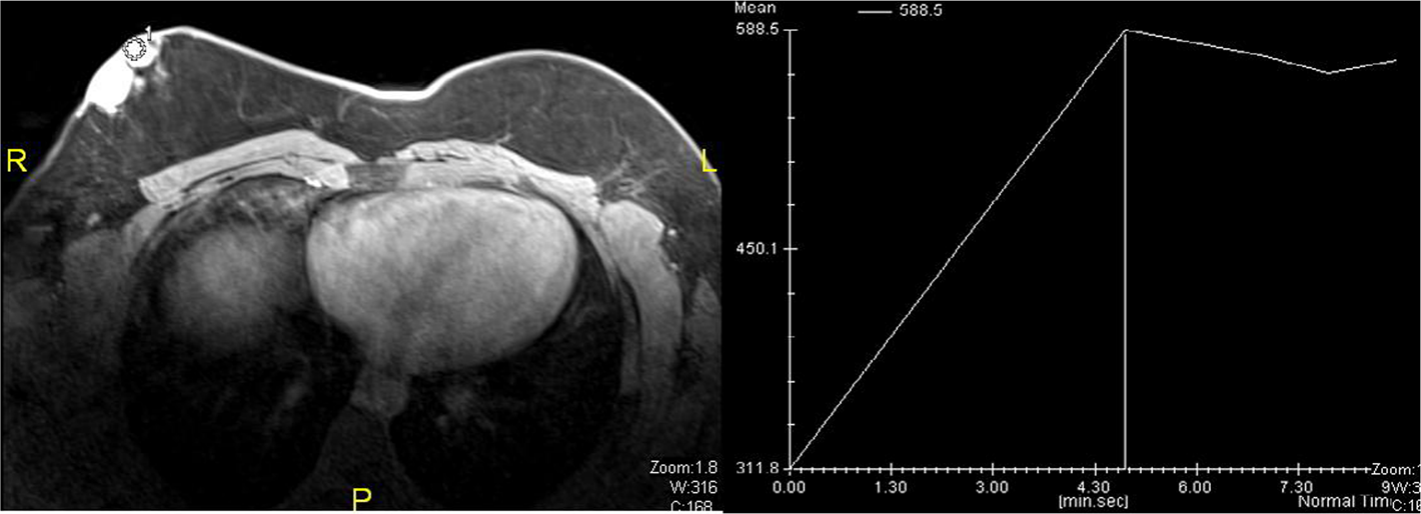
Magnetic resonance imaging showed intense enhancement of intravenous contrast with dye accumulation and washout in the right breast.
